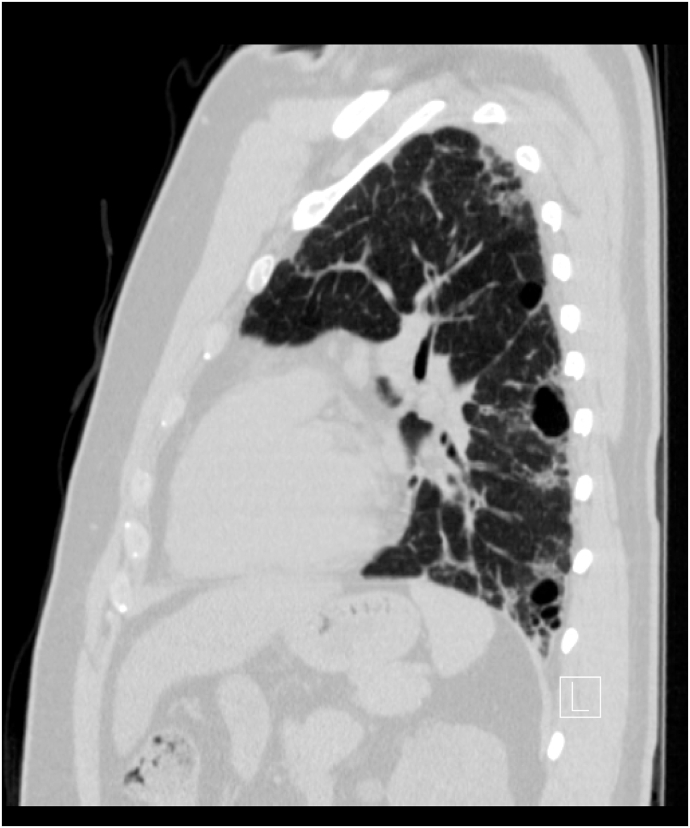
Lateral CT scan view of the chest highlighting left lung volume loss, cystic airspace disease, and fibrosis as well as cystic kidney disease.
